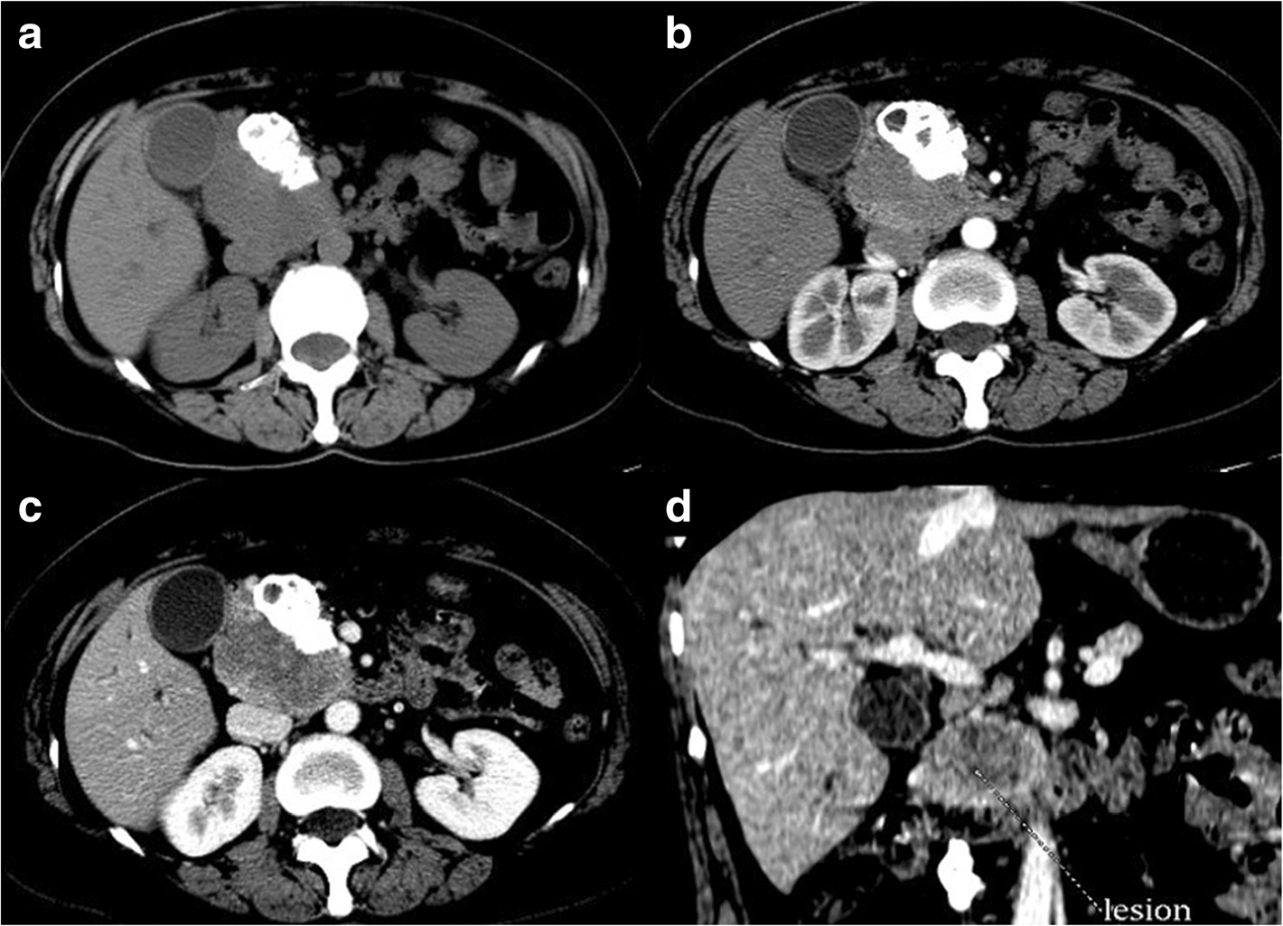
Abdominal CT scans of asymptomatic SPT in a 31-year-old woman. a Unenhanced CT scan showing a 9.0 cm oval mass at the head of the pancreas with homogeneous isoattenuation. Calcification is seen at the periphery of the mass. b Scan obtained during the pancreatic phase. c Scan obtained during the hepatic venous phase showing a mass with a progressive fill-in enhanced pattern. d Coronal pancreatic phase CT image showing complete, smooth, and delayed enhancement of the tumor pseudocapsule
